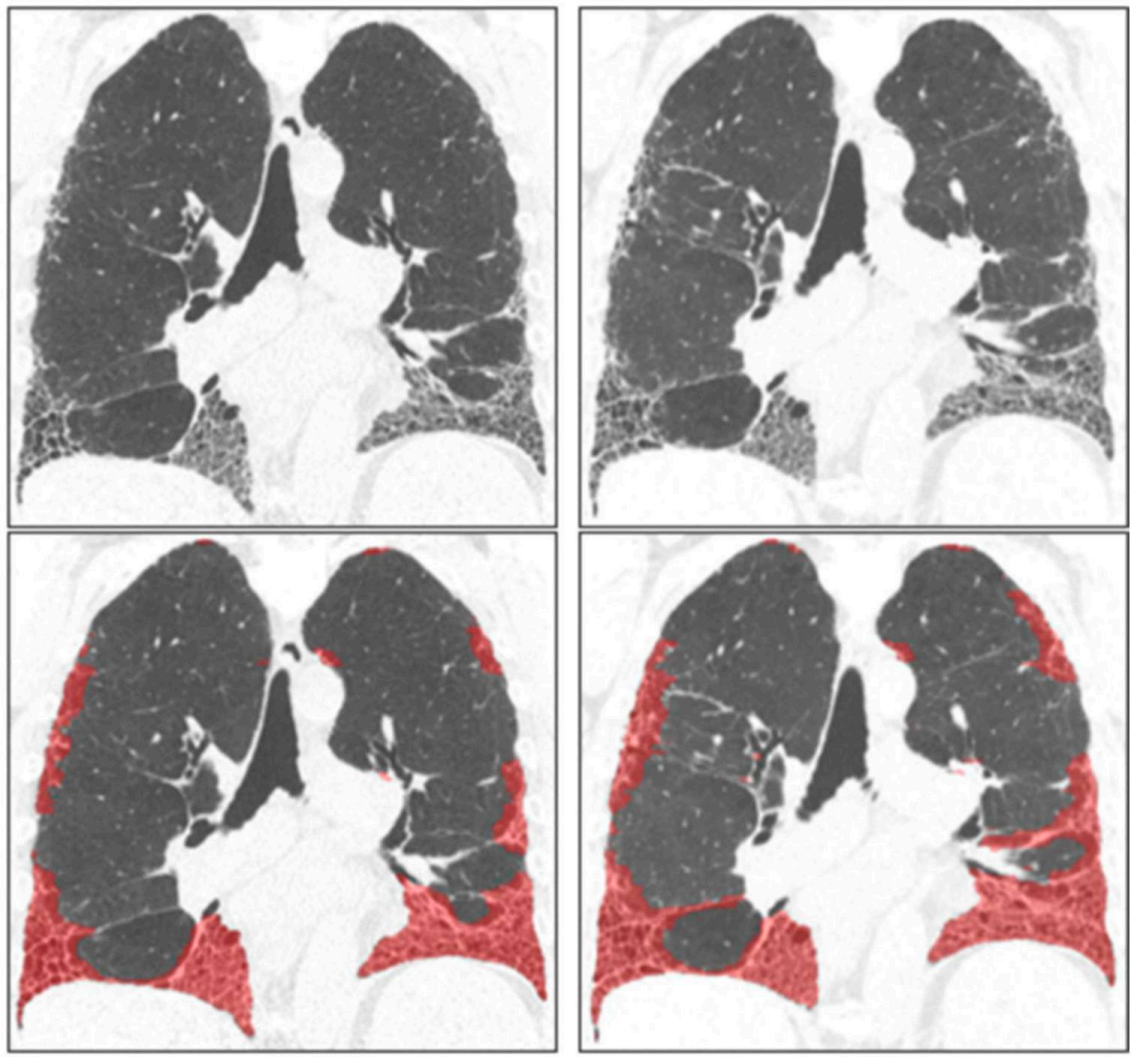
Data-driven texture analysis (DTA) in a patient with IPF at two timepoints. Top left panel is a coronal HRCT reconstruction depicting lower lobe honeycombing and reticulation which is quantified using DTA analysis (red overlay, bottom left panel), baseline FVC was 4.24 L (90.3% predicted), DTA score was 29.0%. On right (upper and lower panels) are coronal HRCT reconstructions in the same patient, 17 months later demonstrating progression of fibrosis. FVC was 4.01 L (86.1% predicted) and DTA score was 39.4%. Courtesy of Prof. Steve Humphries.
